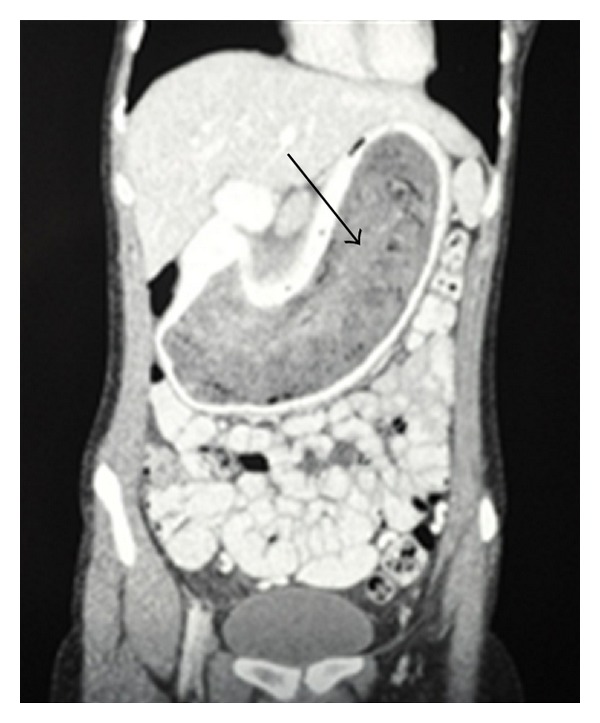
CT scan demonstrating trichobezoar (arrow) occupying the extent of the stomach.
